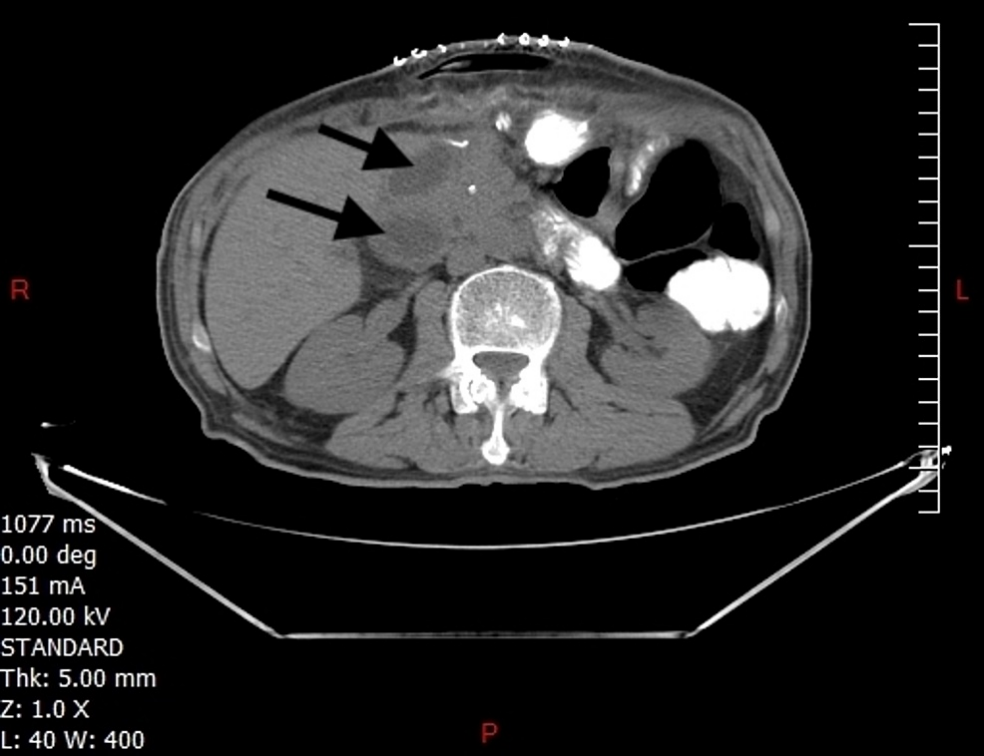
Abdominal CT scan with oral contrast showing biliary ducts dilation (arrows)
